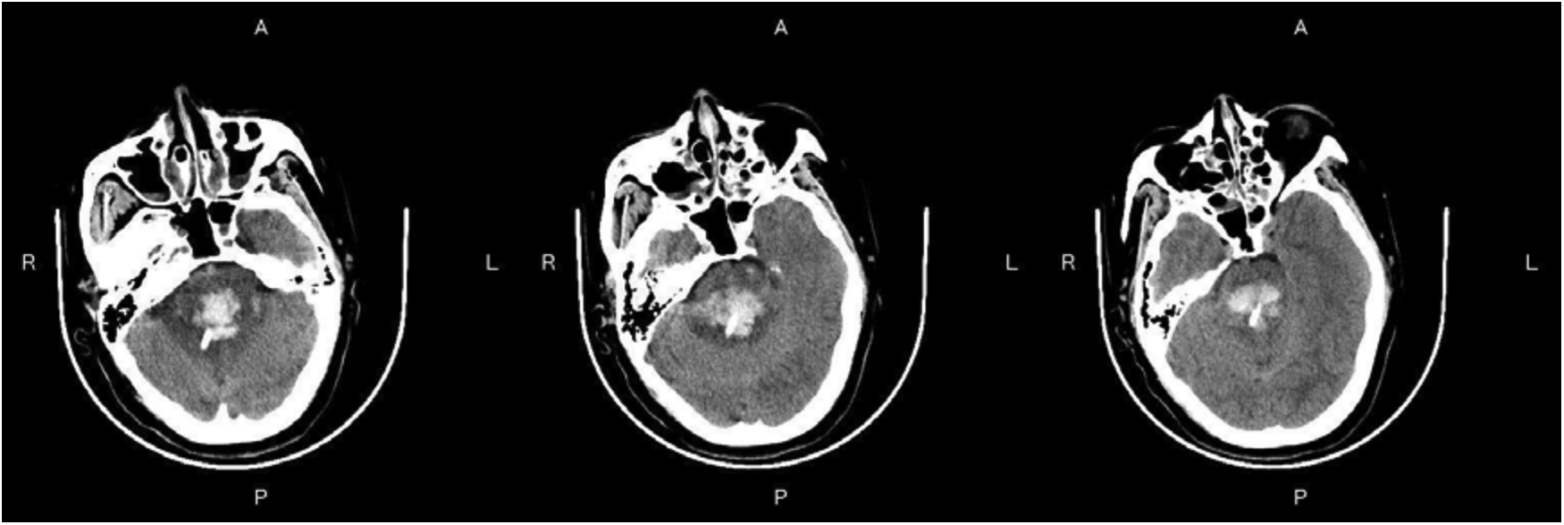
Postoperative imaging: Figure 4 presents a postoperative CT scan of the same patient, obtained 24 h after the stereotactic drainage procedure.
